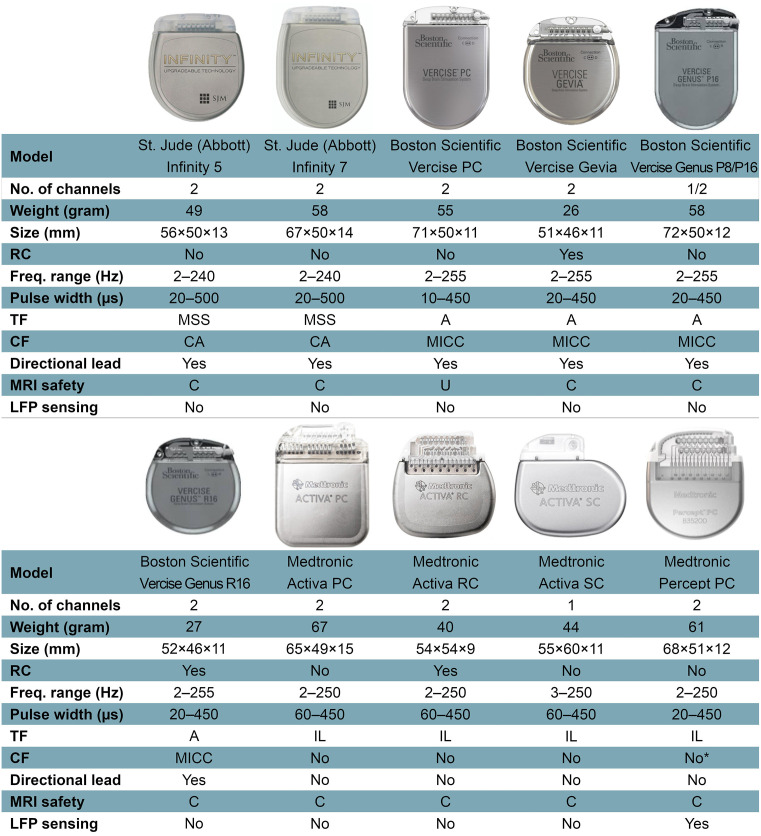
Features of current commercially available internal pulse generators. Abbreviations: A, areas; C, conditional; CA, coactivation; CF, current fractionation; Freq., frequency; Hz, Hertz; IL, interleaving; LFP, local field potential; MICC, multiple independent current control; MRI, magnetic resonance imaging; MSS, multi-stim set; PC, primary cell; RC, rechargeable cell; SC, single cell; TF, temporal fractionation; U, unsafe. *Percept PC can provide independent current control across 16 electrode contacts, but this function is not yet available on physician’s programmer as of March 2021. Not all features or devices are or will be available for a given region and are subject to local regulatory approvals.
